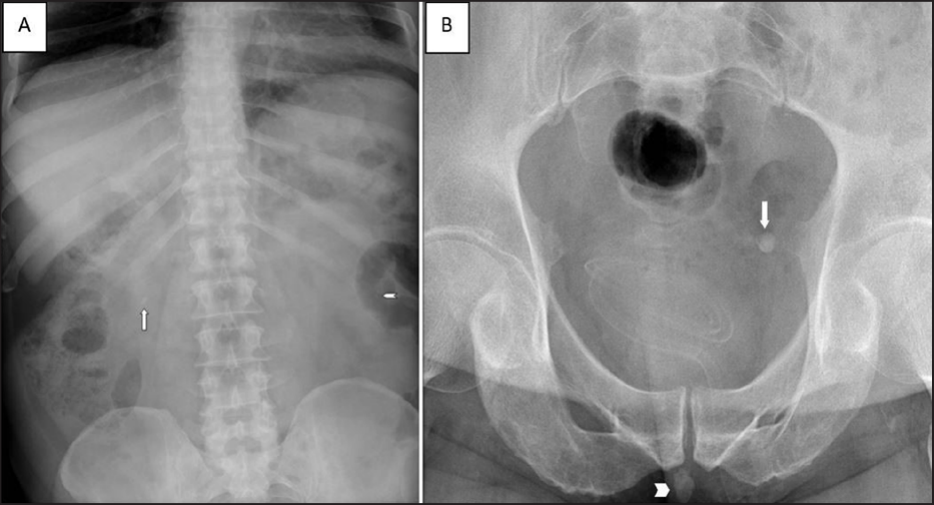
A previously performed CT confirmed the right renal stone (Figure 2A, arrow), a severe hydronephrosis (Figure 2A, arrowhead) of the left kidney due to distal obstructive ureteral stone (Figure 2B, arrow), and a stone at the level of the bulbous urethra (Figure 2C, arrow), which was initially overlooked.
